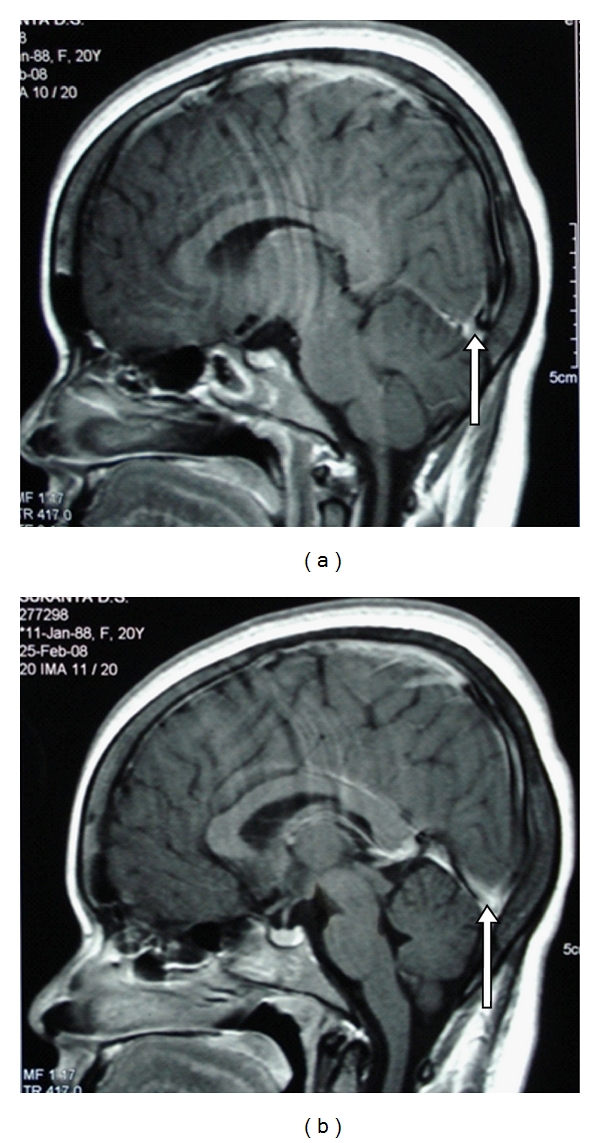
MRI scan showing hyperintensity along the course of vein of Labbe.
